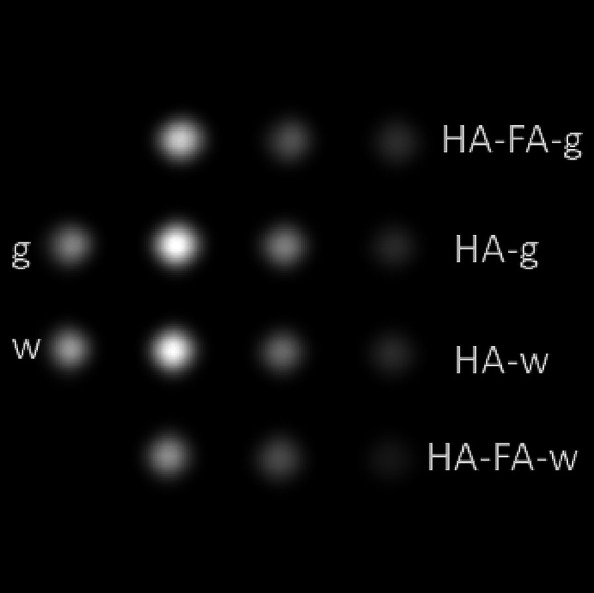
T2-weighted (repetition time 10 s, echo time 7 ms) image of MRI
